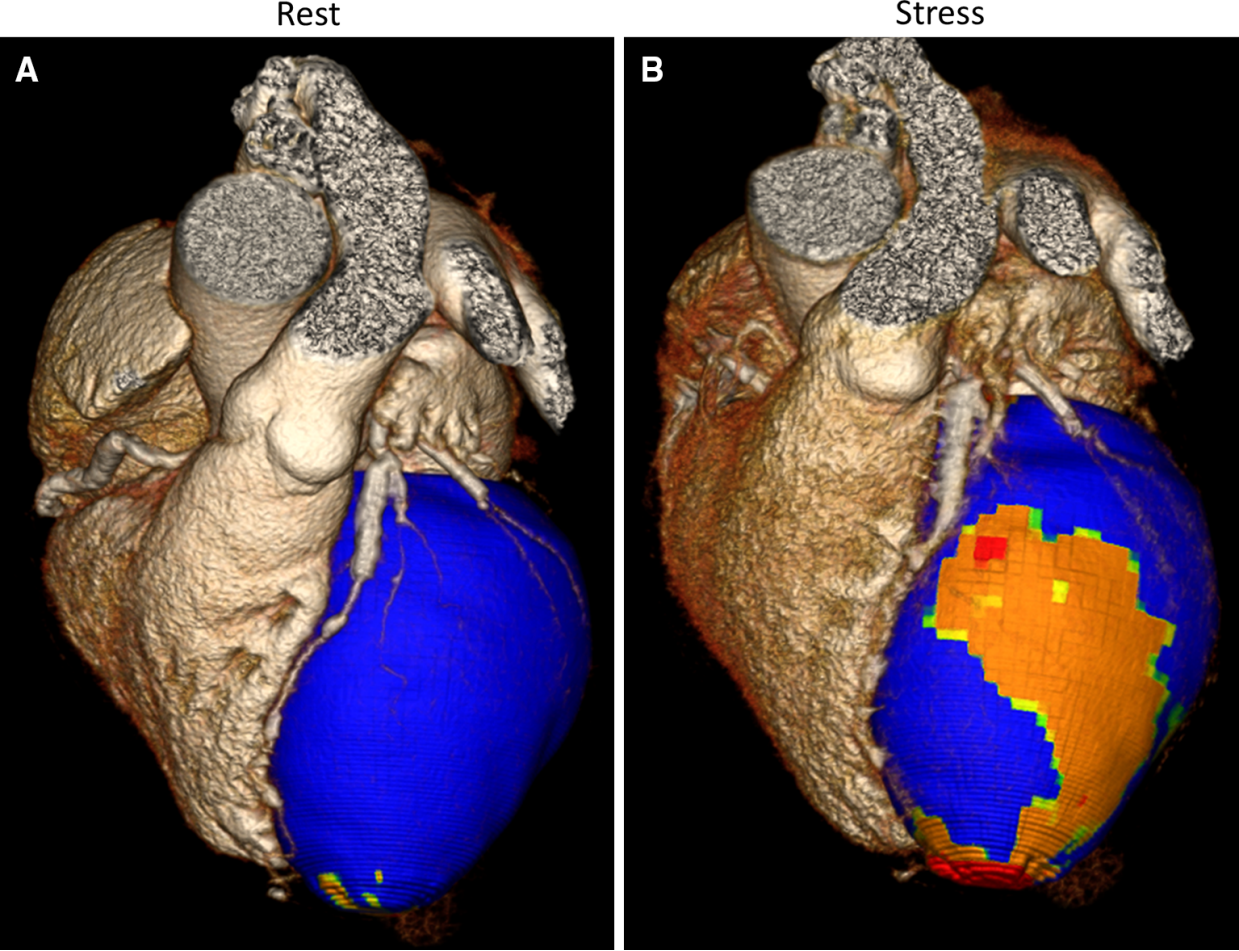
3D fusion of coronary CTA and CTP demonstrating normal myocardial enhancement at rest (A) and myocardial ischemia downstream the D1 at stress (B). *CTA*, coronary computed tomography angiography; *CTP*, myocardial computed tomography perfusion; *D1*, first diagonal branch
